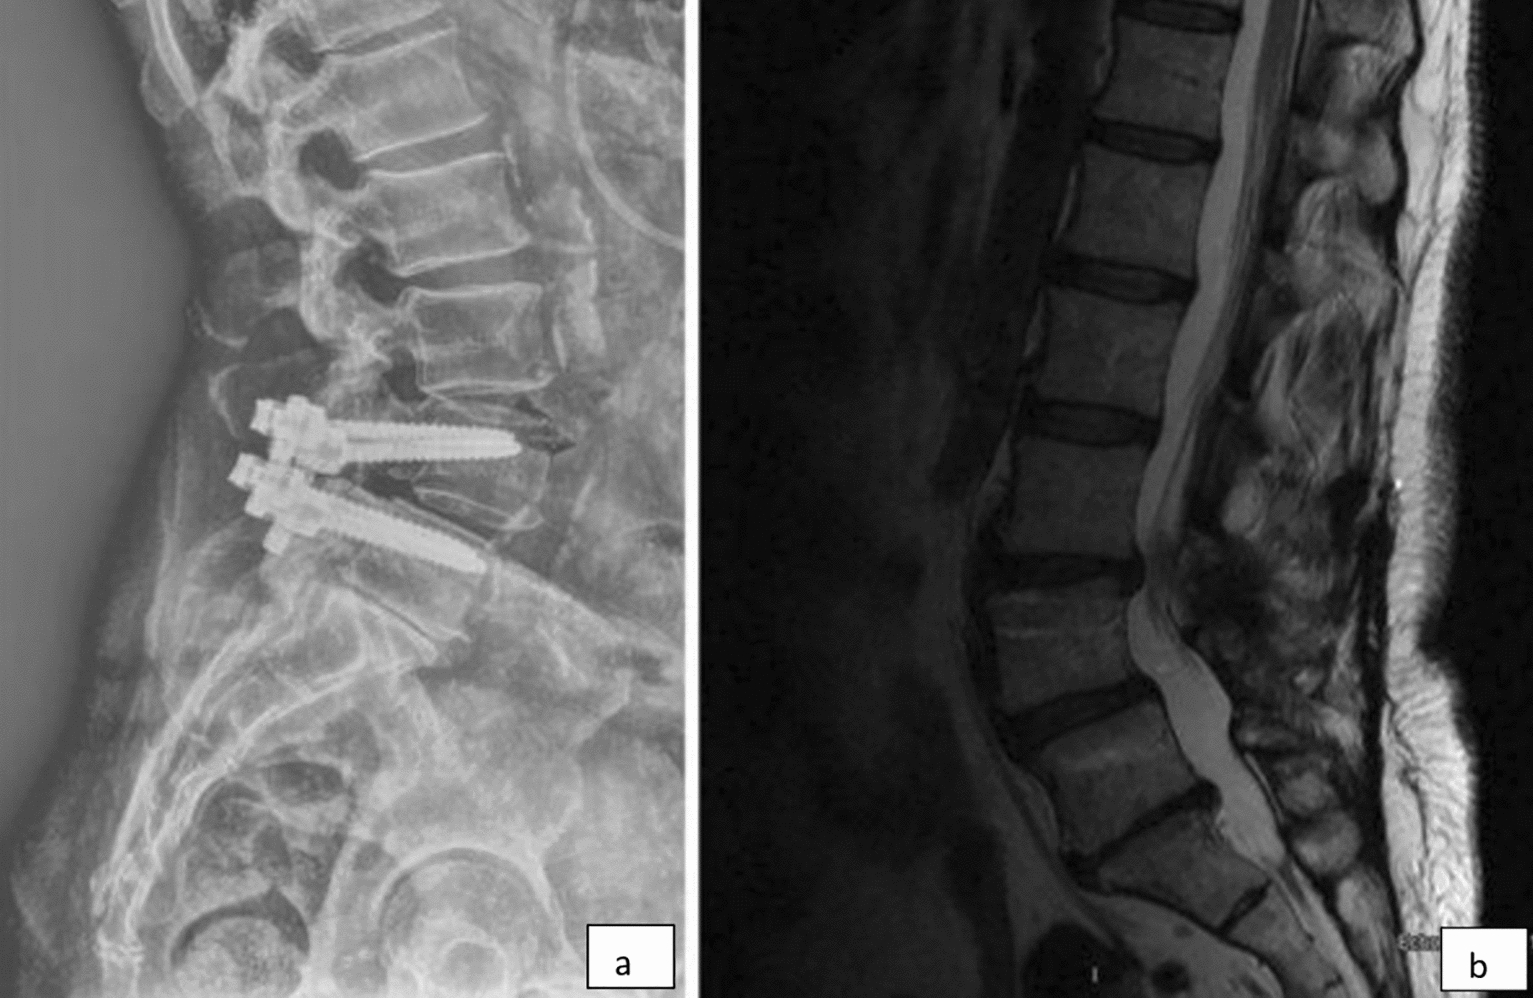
a, b Lateral X-ray and sagittal views of MRI demonstrating adjacent segment disease
